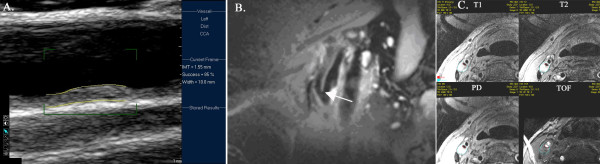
Ultrasound and CMR images. A. B-mode ultrasound of the common carotid artery demonstrating plaque. Automated software detection is used to measure mean posterior IMT. B. Oblique sagittal spin echo of the carotid artery showing the reference flow divider (white arrow). C. Multicontrast CMR T1/T2/proton density spin echo and time of flight gradient echo images of the same axial slice. Luminal/adventitial borders are outlined to measure plaque area/volume.
